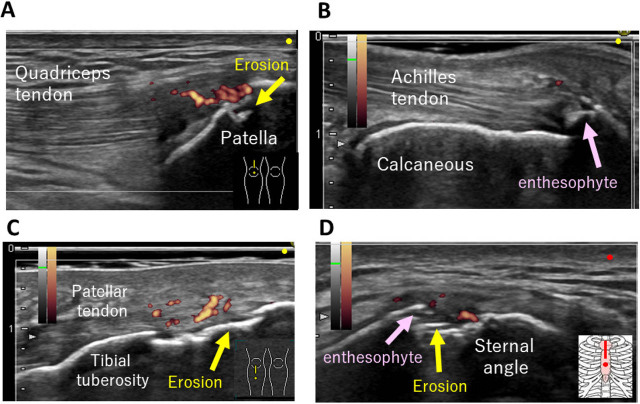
Various enthesitis findings detected by MSUS in SpA patients. (A) Longitudinal view of active enthesitis with power Doppler signals and erosion of the quadriceps tendon in a patient with PsA. (B) Chronic enthesitis with enthesophyte of the Achilles tendon. (C) Patellar tendon active enthesitis from a patient with reactive arthritis. (D) Active enthesitis on the manubriosternal joint in a patient with inflammatory bowel disease–associated arthritis. MSUS, musculoskeletal ultrasonography; PsA, psoriatic arthritis; SpA, spondyloarthritis.
